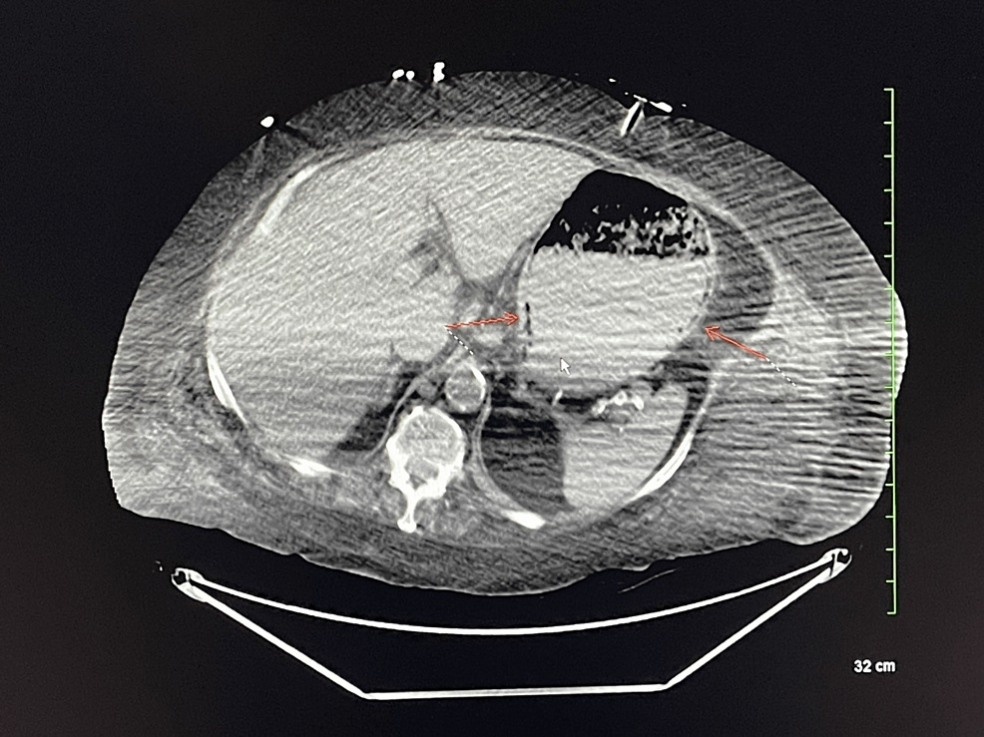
CT of the abdomen slice showing circumferential gas formation (arrows) along the gastric wall CT: computed tomography
